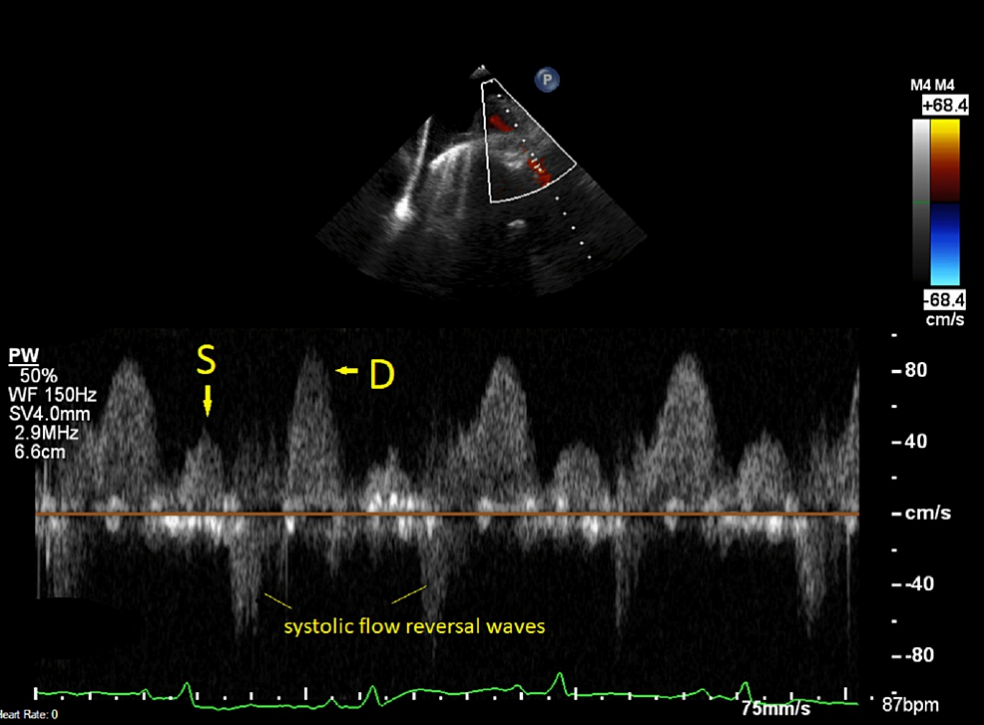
Trans-oesophageal echocardiography. Systolic flow reversal in the right superior pulmonary vein. S = systolic wave; D = diastolic wave
